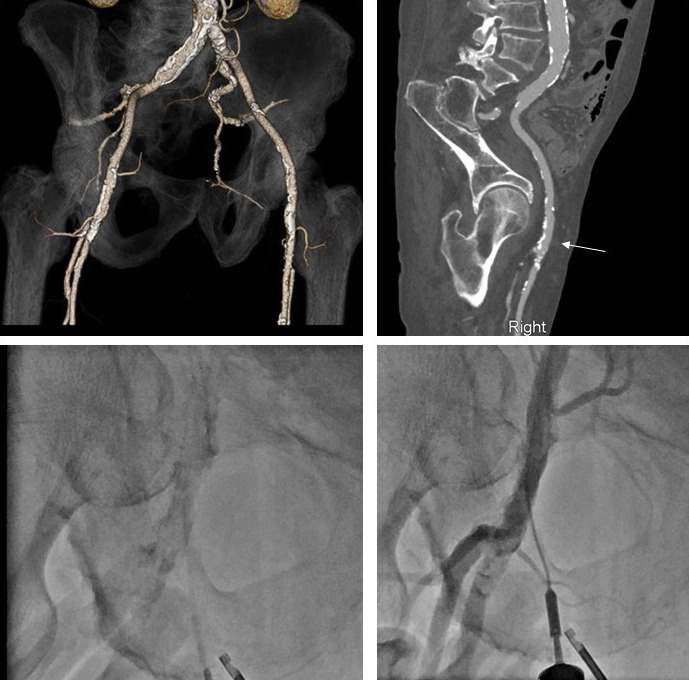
Computed tomography, fluoroscopy, and femoral angiography for
